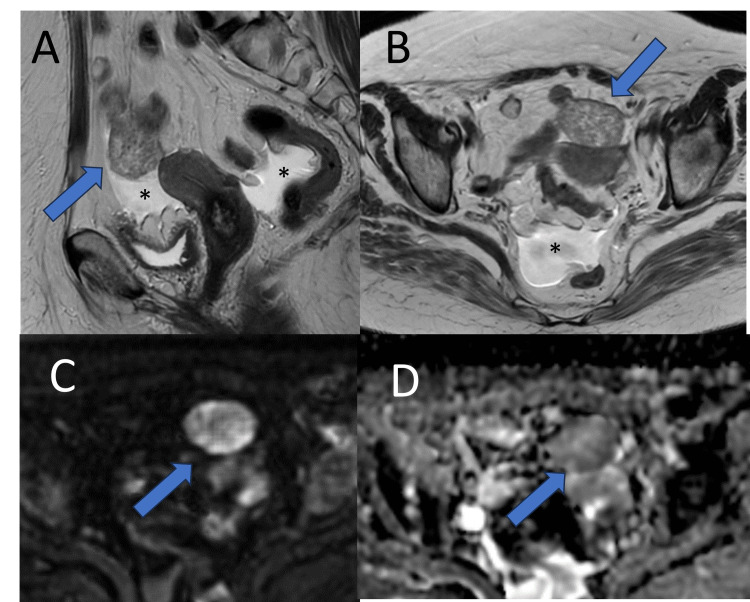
Preoperative MRI (A) Sagittal and (B) axial T2-weighted images demonstrating a well-circumscribed, heterogeneously hyperintense T2 mass (arrows) measuring 5 cm. Clear cleavage planes are identified between the mass and the gynecological organs. Mild pelvic ascites is also present (asterisks). (C) Axial diffusion-weighted image (b-value 1000) and (D) corresponding ADC map of the pelvis. The mass demonstrates restricted diffusion, characterized by high DWI signal intensity and corresponding low ADC signal, suggesting high tissue cellularity MRI: magnetic resonance imaging; ADC: apparent diffusion coefficient; DWI: diffusion-weighted imaging
